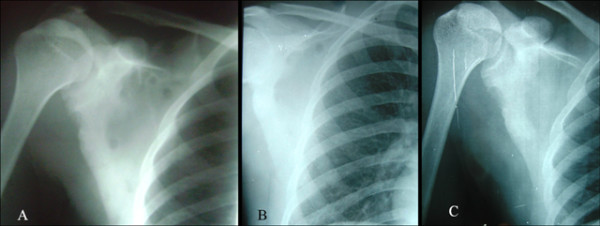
A. Initial radiograph of right scapula (at the time of presentation) showing multiple cystic lesions over the scapular body with surrounding sclerosis B. After 6 months of anti tubercular therapy, most of the cystic lesions healed. Still one cystic cavity is noticed on supero-medial aspect C. After 2 years, the cystic lesions have completely healed.
